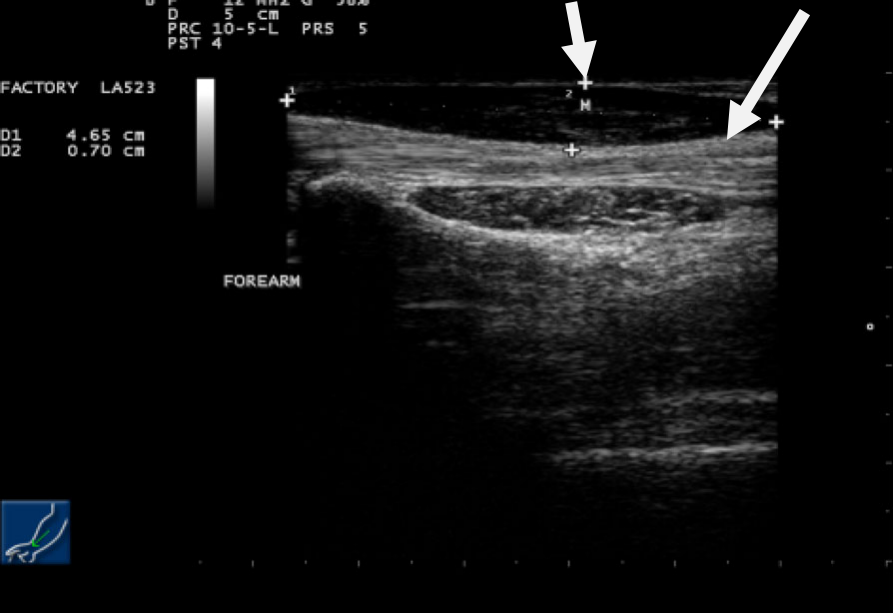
Ultrasonographic image of the right forearm in longitudinal view revealing the muscle mass (the *small arrow* indicates the hypertrophied muscle and the *large arrow* indicates the median nerve)
